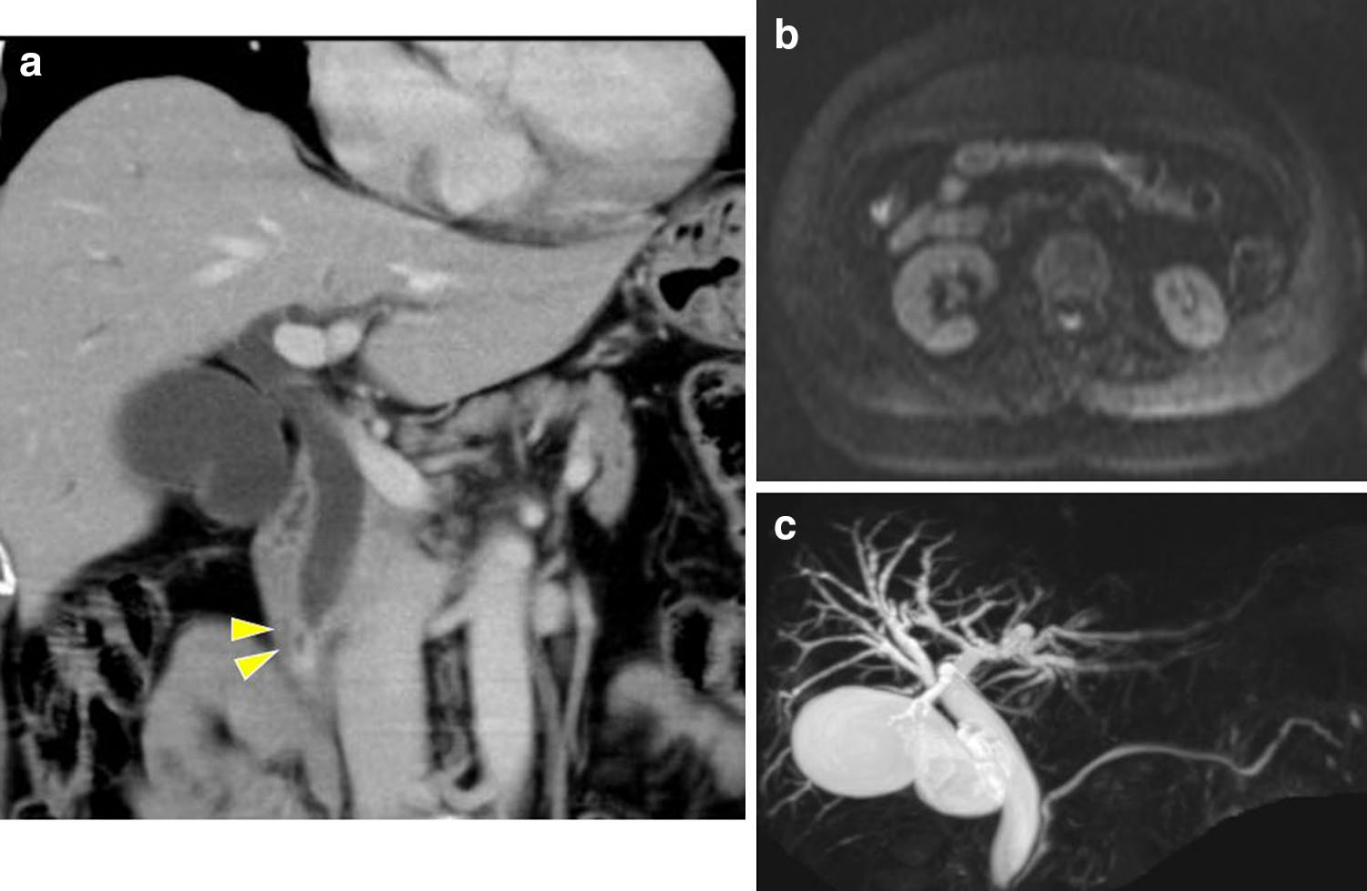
Imaging examinations. a Computed tomography shows a marked dilation of the common bile duct and an enhanced area in the periampullary lesion (yellow arrow head). b Magnetic resonance imaging (diffusion-weighted Imaging) showed no high-signal lesion in the ampulla of Vater. c Magnetic resonance cholangiopancreatography (MRCP) showed no significant main pancreatic duct dilation
